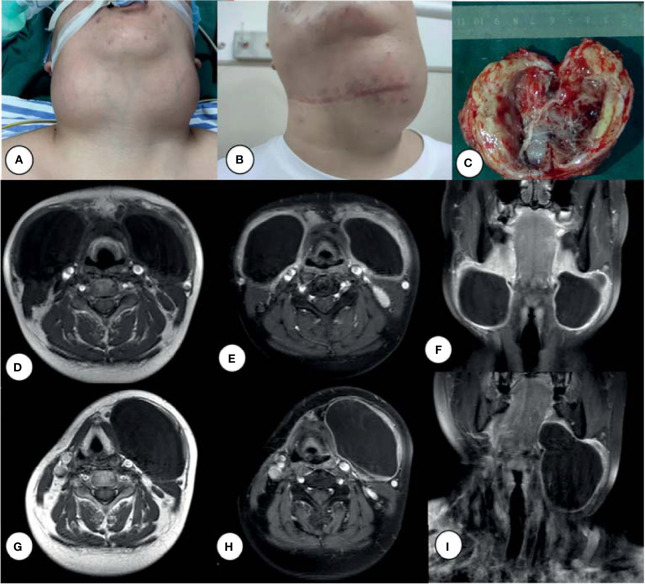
Bilateral cervical giant cysts (A), left cyst enlarged after chemotherapy (B), and surgical excision of the right neck mass (C). MRI of a tumor before surgery (D–F) and after (G–I) chemotherapy: T1-weighted images (D, G), Ga-enhanced T1-weighted images (E, F, H, I).
